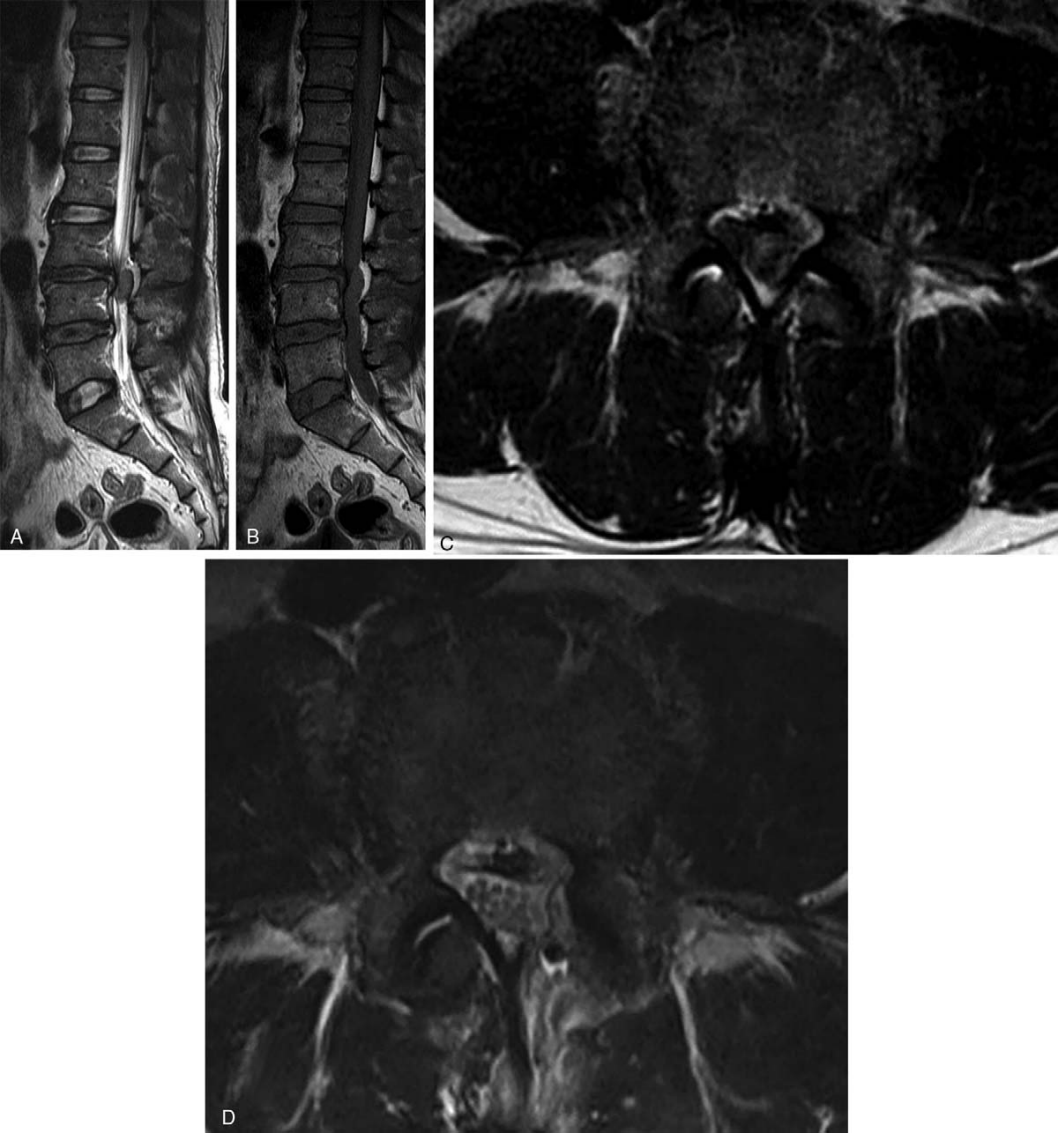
Preoperative sagittal T2-weighted magnetic resonance imaging (MRI) (A), sagittal T1-weighted MRI (B), and axial T2-weighted MRI (C) of the lumbar spine demonstrated an extradural mass lesion in the left-lateral and posterior epidural space at the level of L3-L4 with severe thecal sac compression (white arrow). Postoperative axial T2-weighted MRI (D) showed laminectomy and no thecal sac compression.
